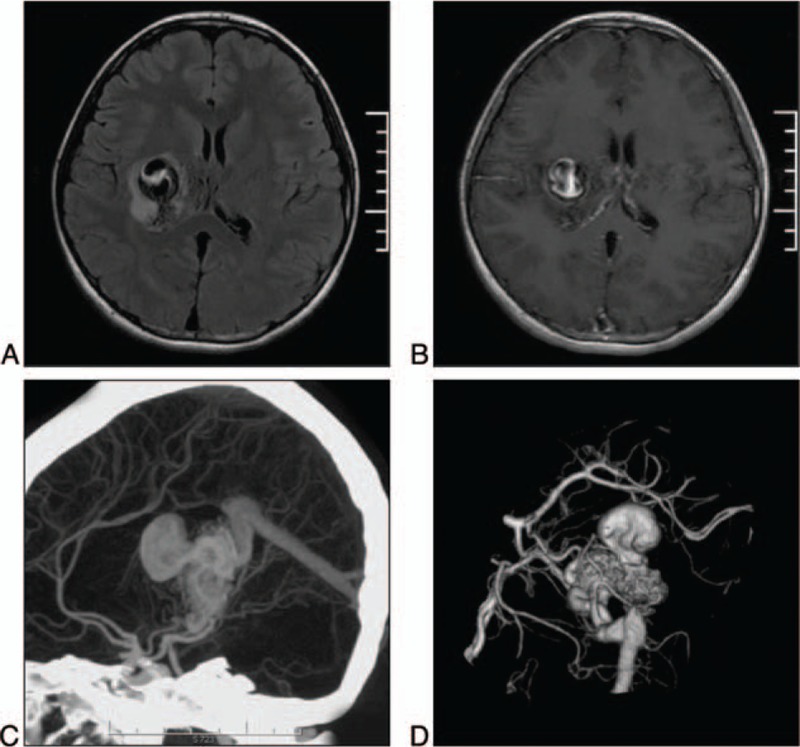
A: The FLAIR sequence on MRI shows a linear and round flow void and perilesional edema in the region of the right basal ganglia, indicating a BAVM. A signal with mixed intensity is noted in the round lesion, in accordance with the formation of an intraluminal thrombosis. B: Gadolinium-enhanced T1 sequence shows peripheral enhancement of the round lesion. C-D: MIP and 3-D CTA showing that the BAVM is fed by the ipsilateral PCA and AchA and drains into the vein of Galen. A large varix is also noted at the top of the BAVM. AchA = anterior choroidal artery, BAVM = brain arteriovenous malformation, CTA = computed tomography angiography, FLAIR = fluid attenuation inversion recovery, MIP = maximum intensity projection, PCA = posterior cerebral artery.
